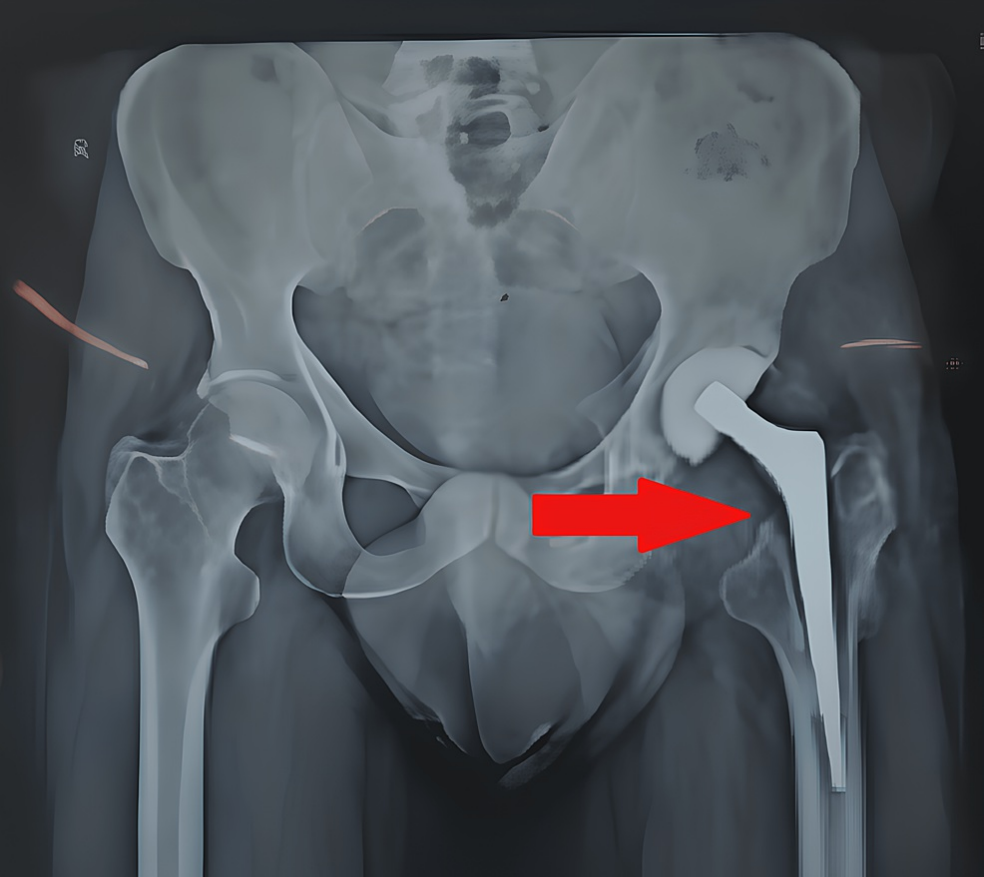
Postoperative X-ray after cemented implant (August 11, 2021)
